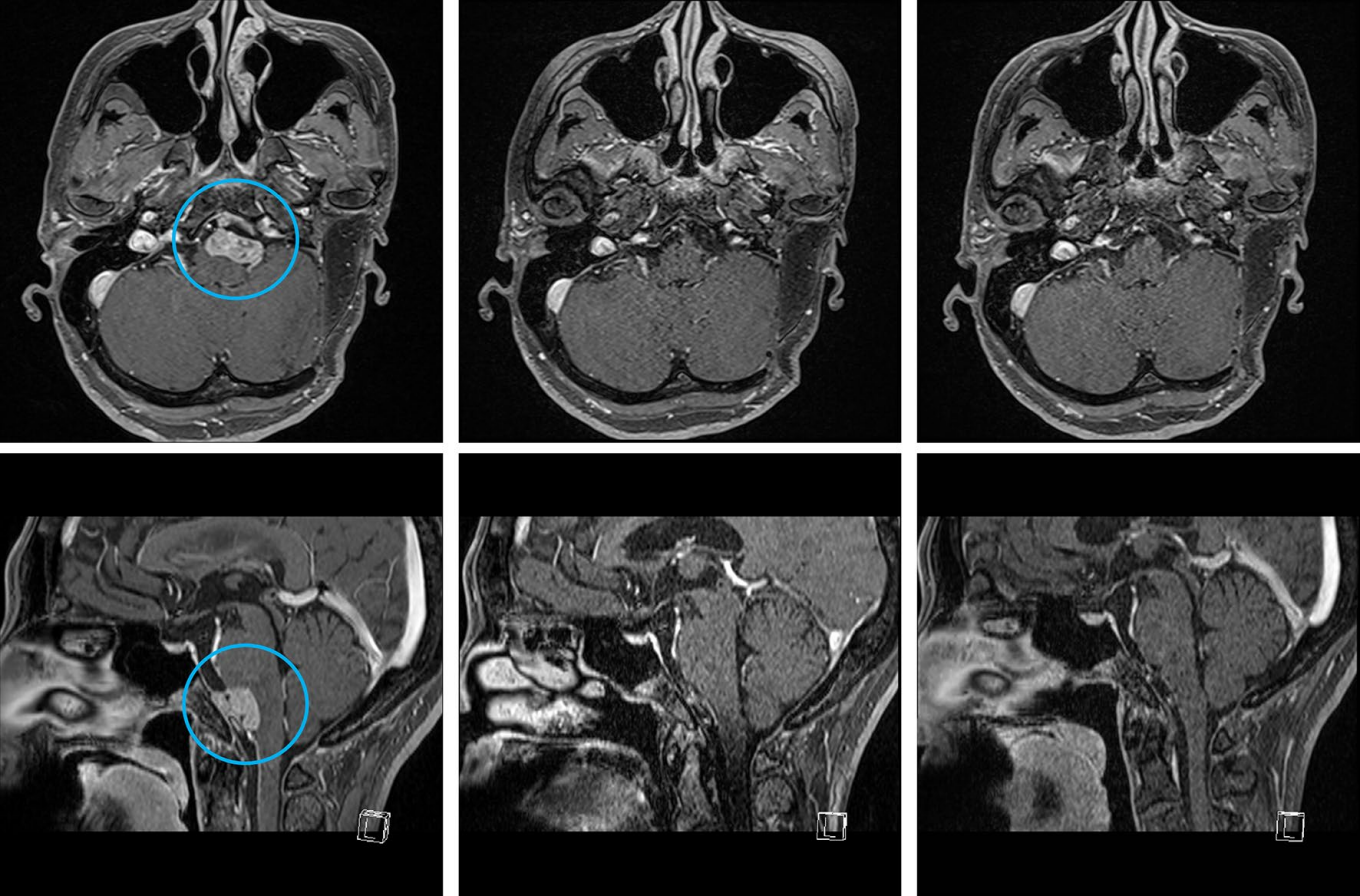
Gadolinium-enhanced T1-weighted magnetic resonance imaging (MRI). Axial (upper) and sagittal (low) MRI show that the tumor (circle) had extended to the left anterior of the medulla oblongata at baseline (left), remarkable regression at 3 weeks (middle), and complete disappearance at 12 weeks after the start of treatment with larotrectinib (right)
